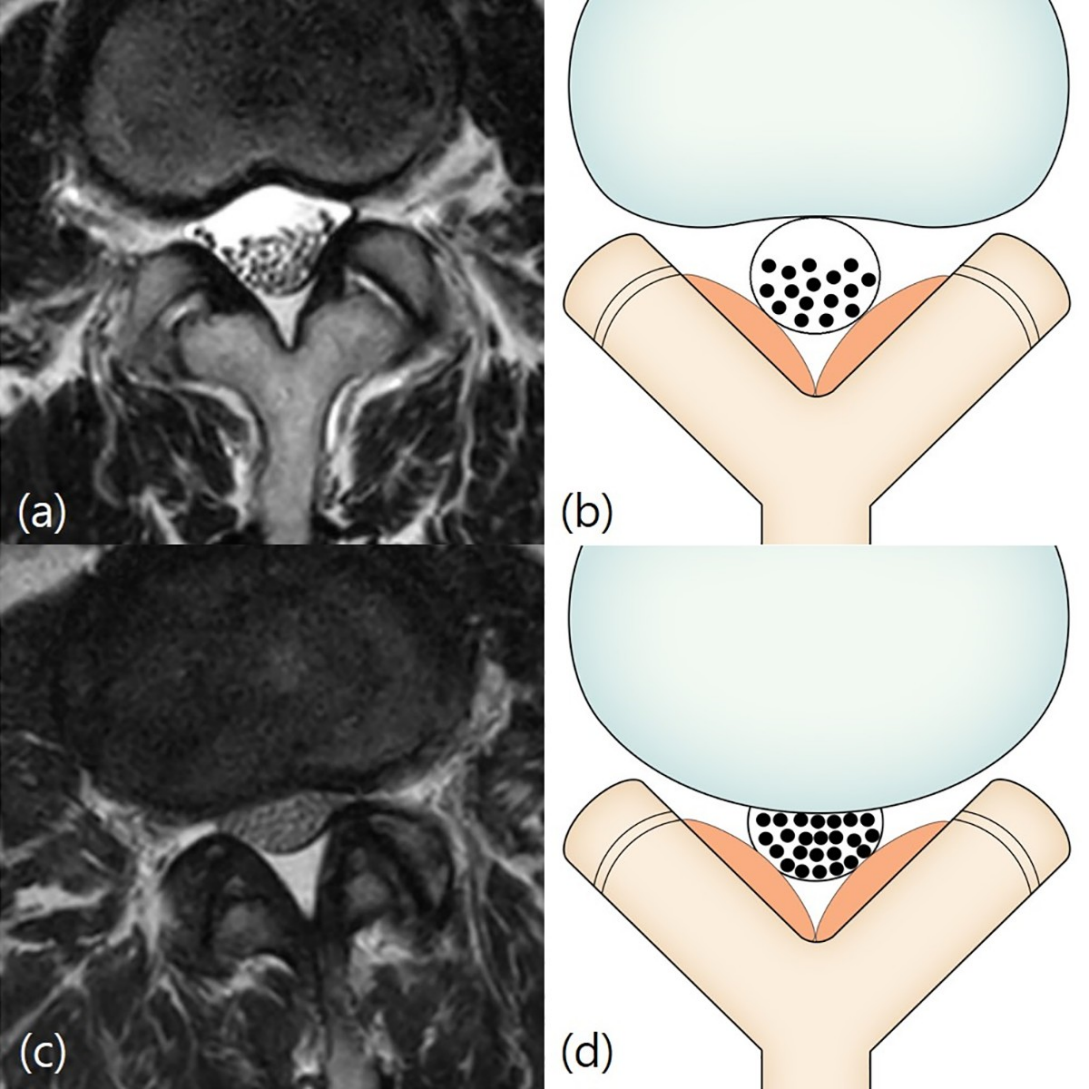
Lee classification. Grade 0 and 1 (no or mild stenosis). Figs 4A and 3B: Grade 0 (no stenosis). A 66-year-old woman with lower back pain. T2-weighted axial magnetic resonance image at L2/3 disc level shows no obliteration of the anterior cerebrospinal fluid space. Fig 4C and 4D: Grade 1 (mild stenosis). A 61-year-old woman with lower back pain. T2-weighted axial magnetic resonance image at L2/3 disc level shows mild obliteration of the anterior cerebrospinal fluid space and all cauda equina clearly separated from each other.
